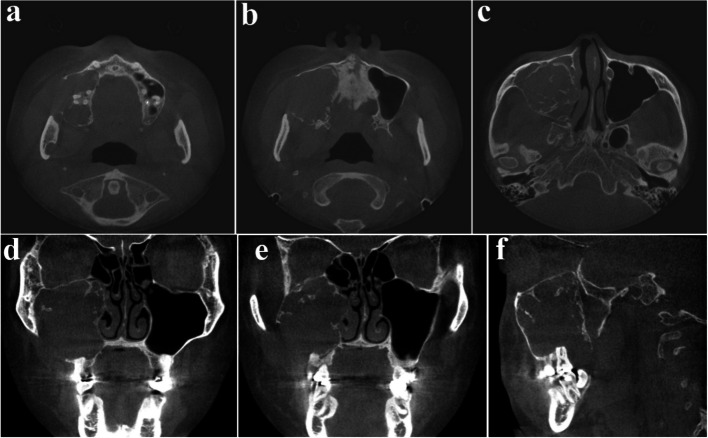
CT image showing extensive bone destruction from the maxillary alveolar ridge to the orbital floor with cortical expansion. a axial view, near the alveolar ridge, (b) axial view, maxillary sinus, (c) axial view, near the orbital floor (d) coronal view, premolar area, (e) coronal view, molar area, (f) sagittal view
